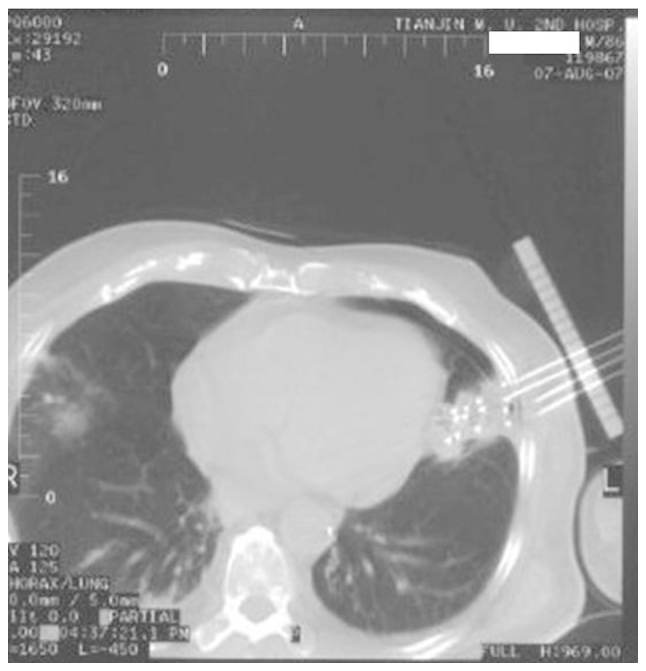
CT-guided ^125^I seed brachytherapy.
